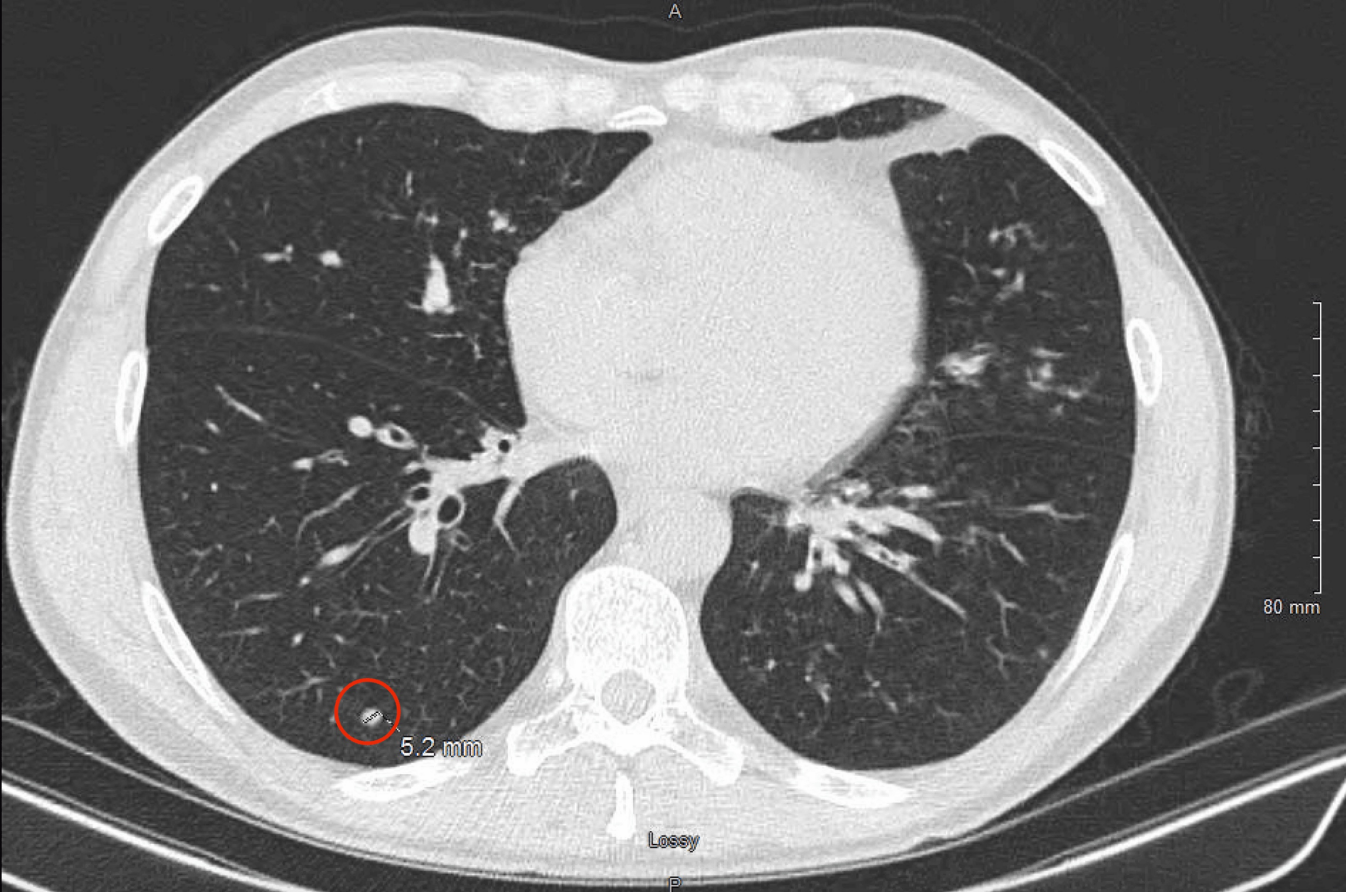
Chest CT prior to treatment initiation showing a pulmonary nodule A chest CT prior to treatment initiation shows multiple pulmonary nodules. This slice highlights a 5.2 mm nodule in the right posterior lower lobe (red circle).
